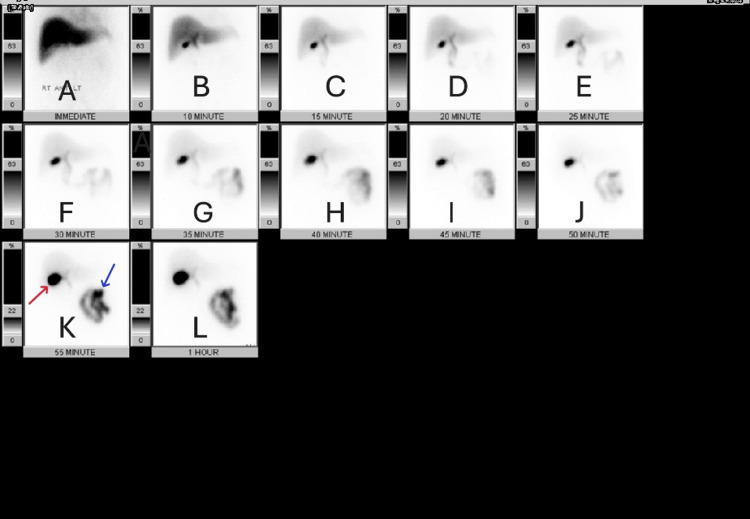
Dynamic hepatobiliary scintigraphy demonstrated impaired gallbladder filling and contraction Hepatobiliary scintigraphy study demonstrated normal hepatic uptake and prompt biliary excretion with tracer passage into the small bowel (Frames A-L); the gallbladder (red arrow); the small intestine (blue arrow); and Frames K and L showed no contraction of the gallbladder.
